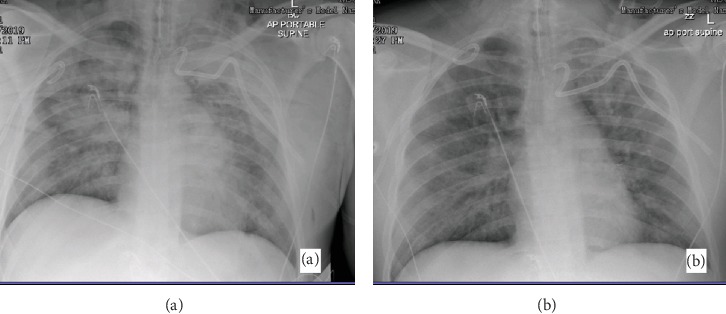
(a) Chest X-ray preprone positioning showing bilateral air space disease with patchy bilateral consolidation from massive spillage of blood from bleeding left bronchial varix and atelectasis. Bilateral pneumothoraxes with placement of bilateral chest tubes. (b) Forty-eight (48) hours post prone positioning with mechanical clot extraction showed interval improvement of airspace disease.
